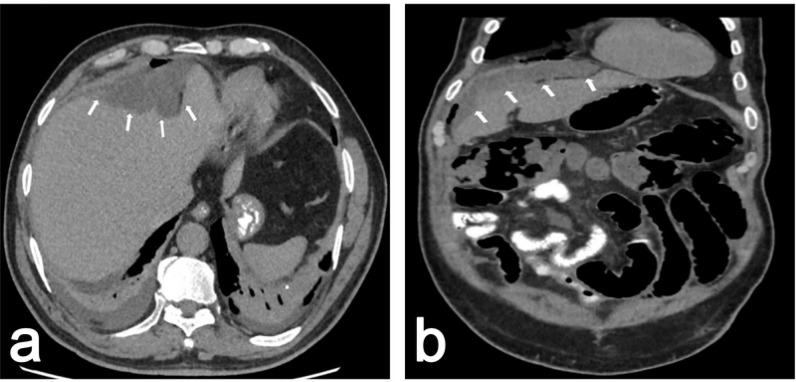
a: Axial computed tomography (CT) scan view of the abdomen, with the cystic mass compressing the liver and no pathologic contrast enhancement. The arrows show the margins of the tumor. b: Coronal CT scan view of the abdomen, with the cystic mass (4.7 × 11 × 10 cm) located at the subdiaphragmatic area adjacent to the liver, with no pathologic contrast enhancement. The arrows show the margins of the tumor.
